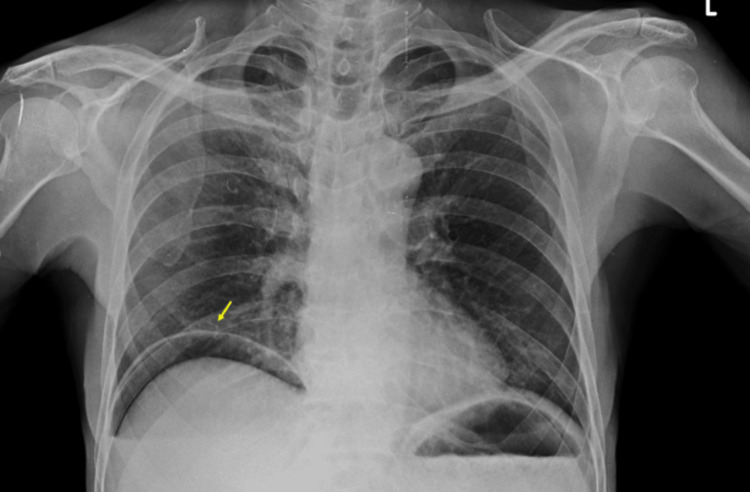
Chest radiograph frontal view showing air under the right diaphragm (yellow arrow), evident of pneumoperitoneum.
